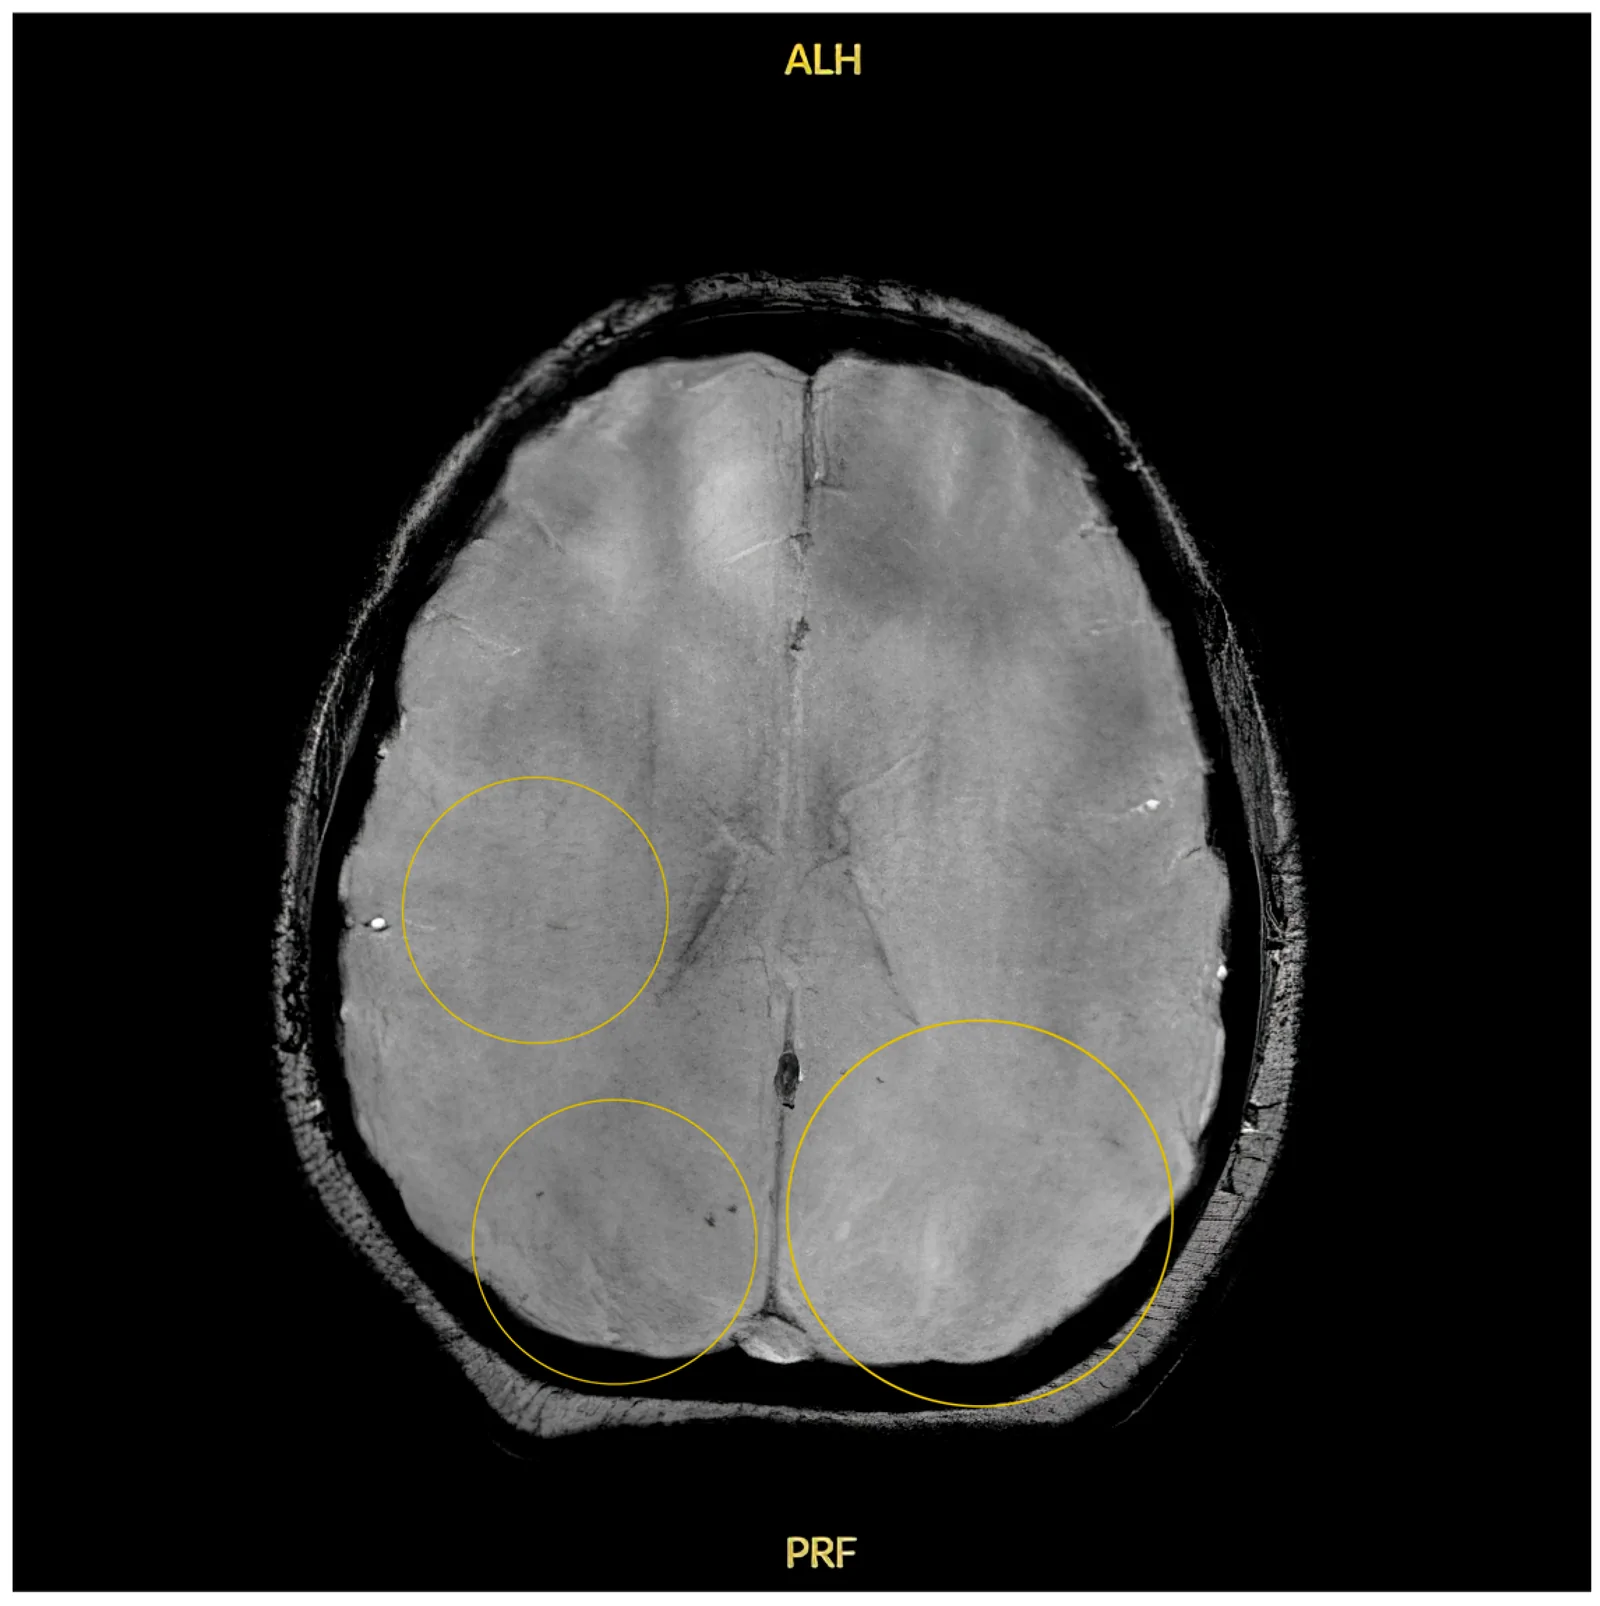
Brain magnetic resonance imaging demonstrates multiple cerebral microhemorrhages involving the bilateral parieto-occipital deep white matter in the setting of severe DKA, uremia, hypertensive crisis, and multifactorial encephalopathy.
